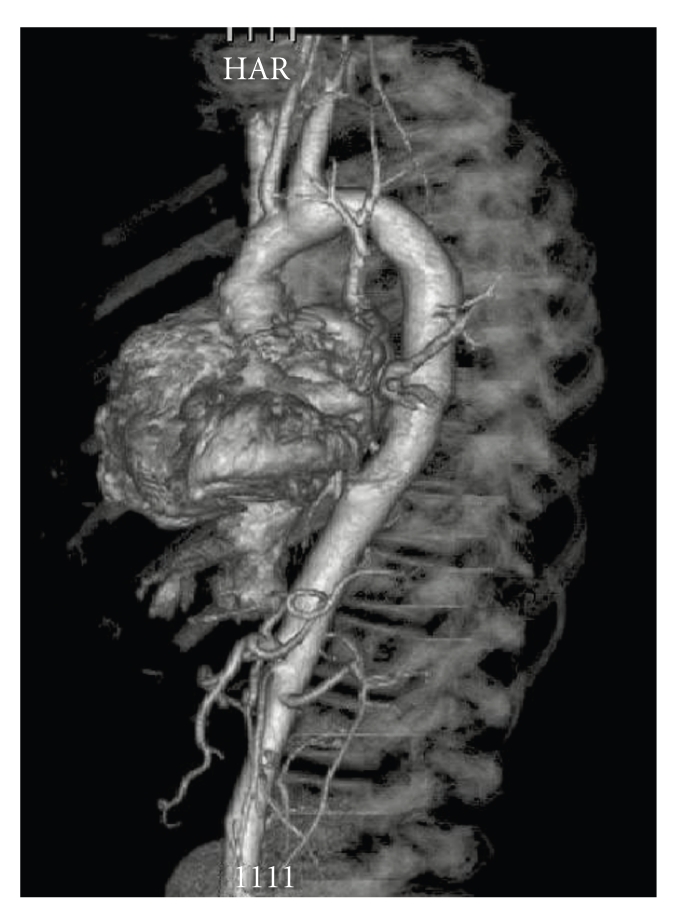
Postoperative CT angiography.
